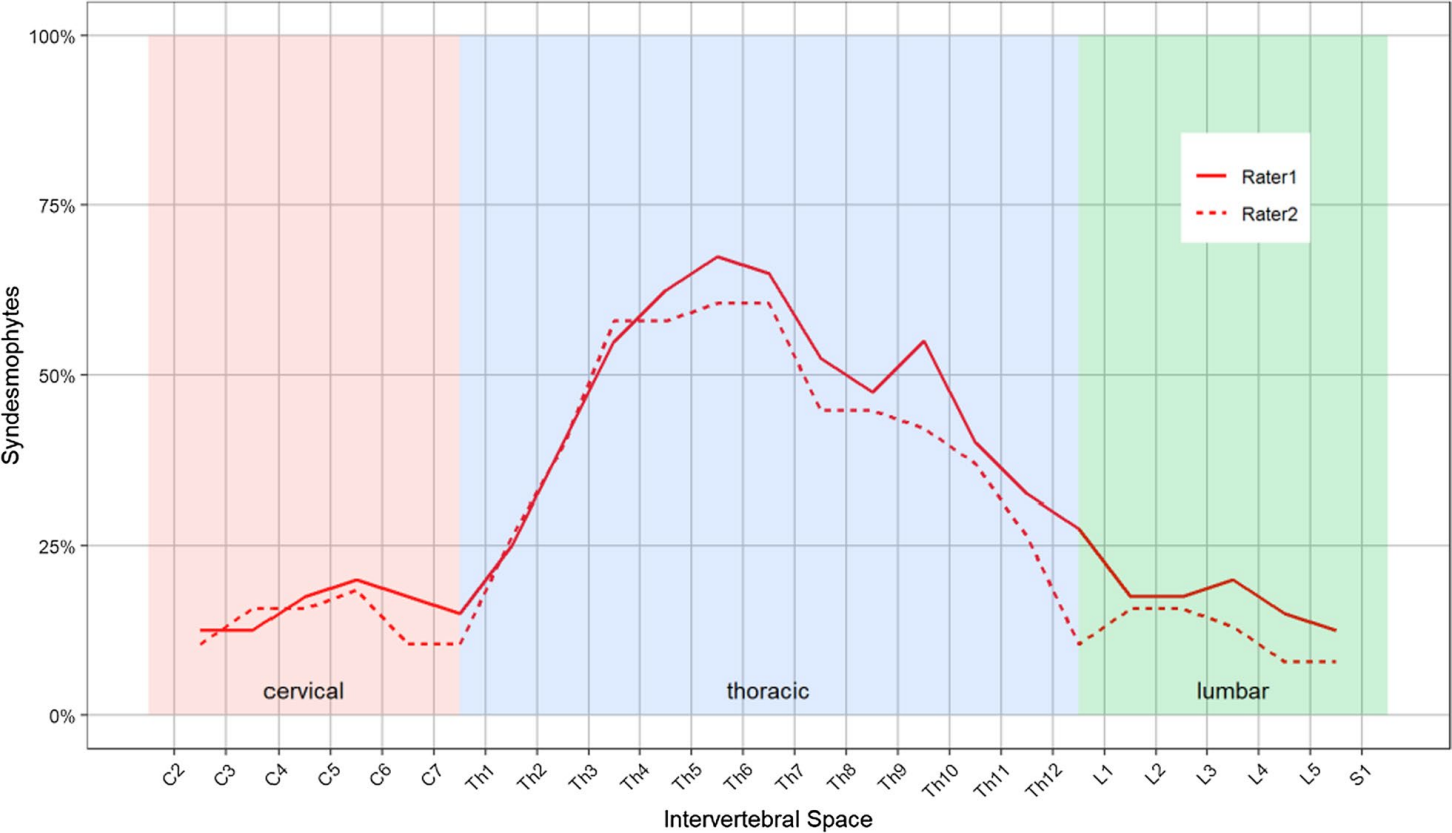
Frequencies of anterior vertebral syndesmophytes diagnosed with MRI. Syndesmophytes reported on MRI were identified most frequently in the thoracic spine, a vertebral portion that is not considered with mSASSS
